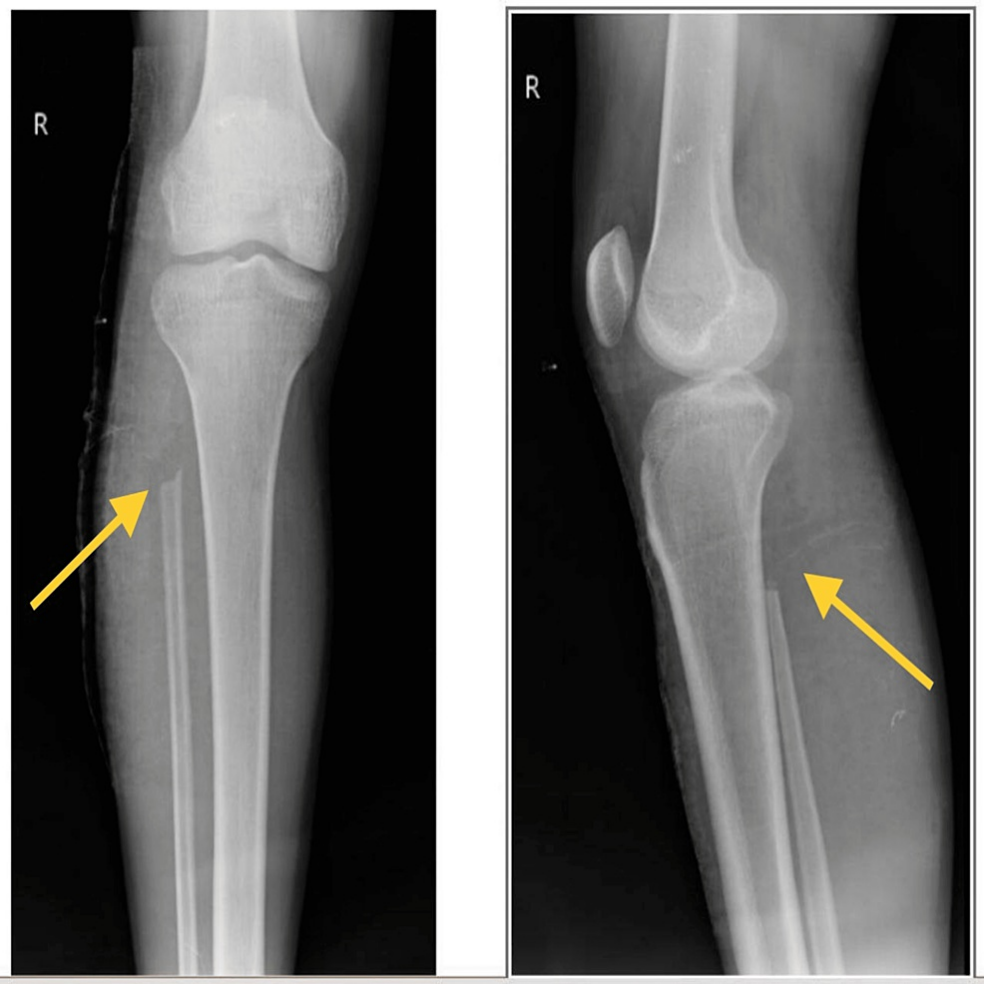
Postoperative X-ray (anteroposterior and lateral view) of the right knee joint with proximal tibia and fibula showing absent fibular head (arrows) as it was excised in the en bloc resection of the cyst.
